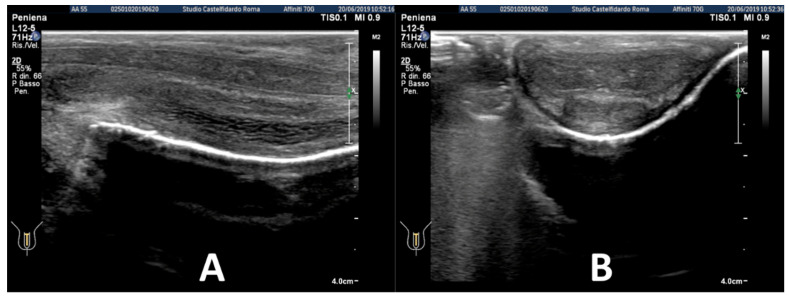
Ultrasonography of the penis after the fourth treatment cycle. (A) longitudinal view, (B) transverse view.
